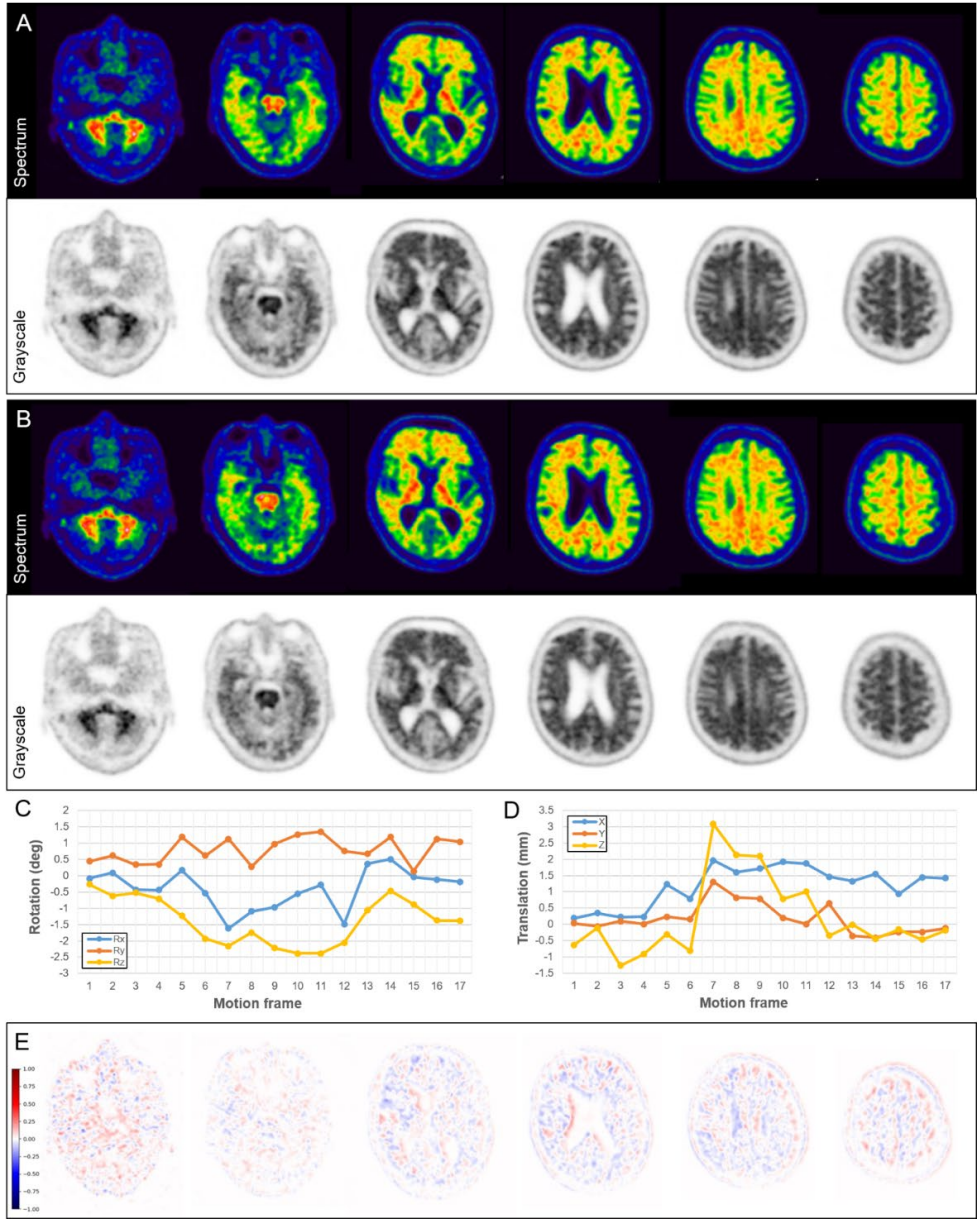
^18^F-flutemetamol brain PET study in a 79-year-old man. Both readers interpreted both conventional (A) and motion-corrected (B) PET images as amyloid positive with good image quality. However, the motion correction algorithm detected 17 subtle head movements with rotation changes of up to 2.39° (C) and translations of up to 3.09 mm (D) relative to the initial position. In quantitative analysis, the UR differed by 0.12 between the conventional and motion-corrected PET images. The difference in normalized SUV between both PET images was identified in the difference map by subtracting the conventional from the motion-corrected PET images (E)
